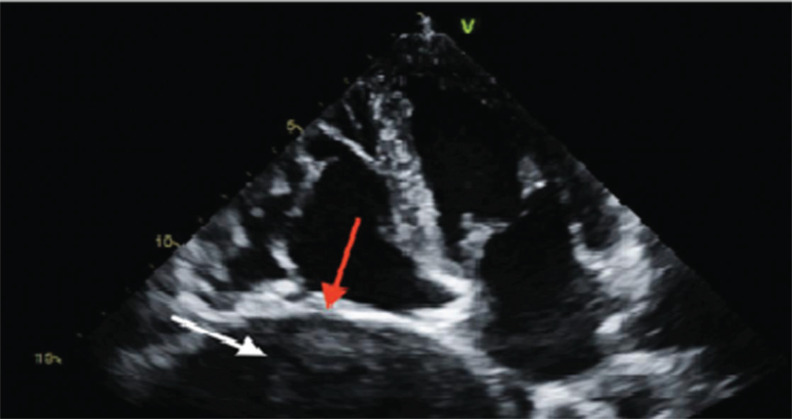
Transthoracic echocardiogram showing lung mass.
